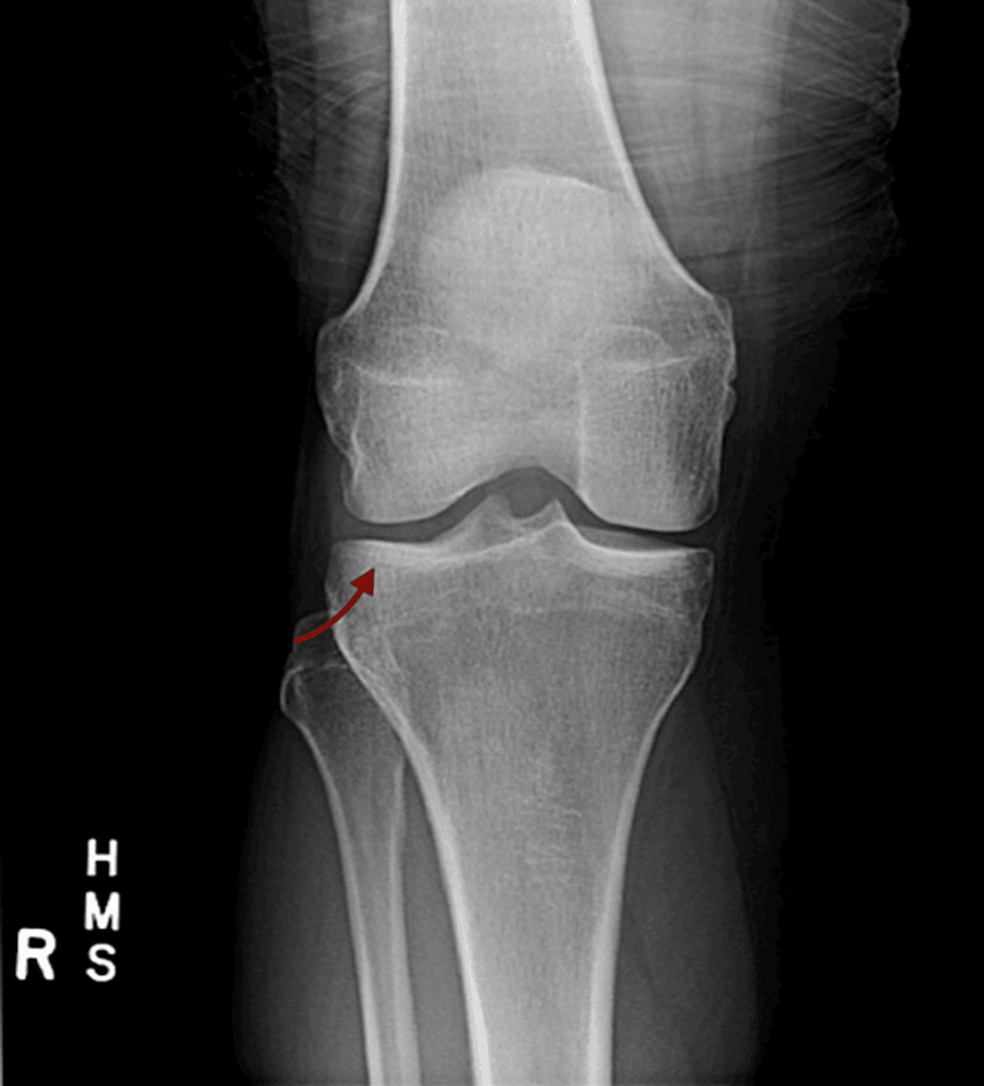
Posteroanterior view X-ray of the right knee Arrow indicates no concerning findings for seronegative rheumatoid arthritis (RA) such as narrowing of joint space and erosion of joints.
